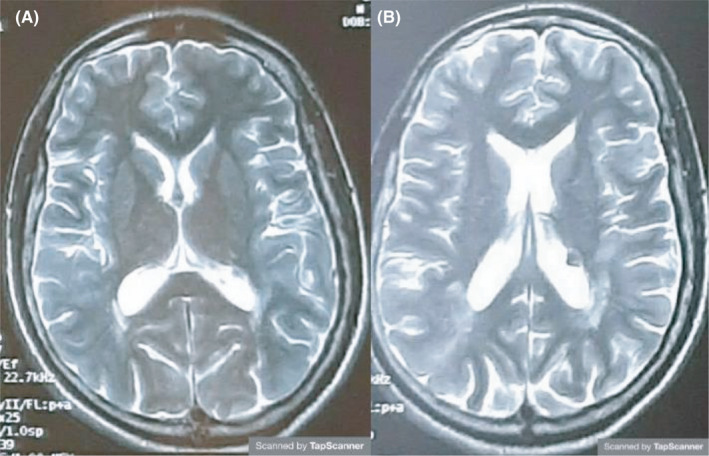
Previous (A) and current (B) magnetic resonance images of the brain. This figure shows cortical‐subcortical tuberous and subependymal nodules without any overt radiological aspect modification within the time course of the illness
